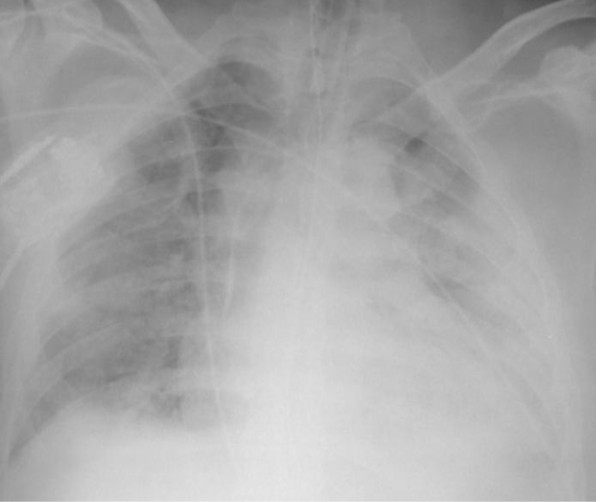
X-ray: bilateral pulmonary infiltrates (ARDS).
